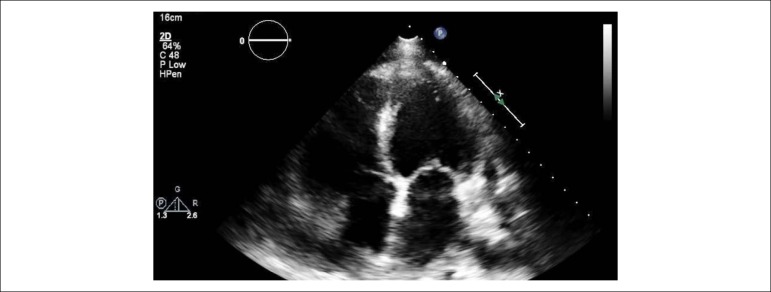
Transthoracic echocardiogram - apical windows. Link: http://publicacoes.cardiol.br/portal/abc/portugues/2020/v11402/dor-abdominal-uma-apresentacaoincomum-de-ruptura-miocardica.asp
